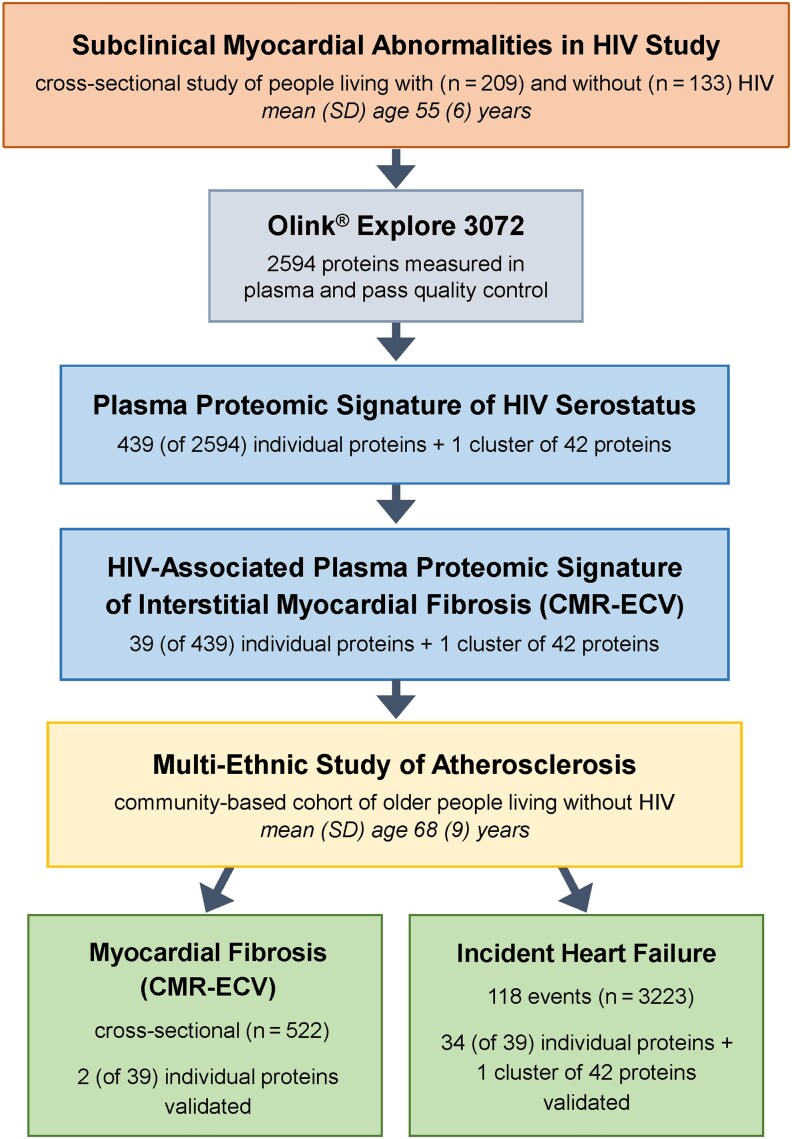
Study overview and analytic flow. Abbreviations: CMR-ECV, myocardial extracellular volume fraction ascertained by cardiovascular magnetic resonance imaging; HIV, human immunodeficiency virus; SD, standard deviation.
